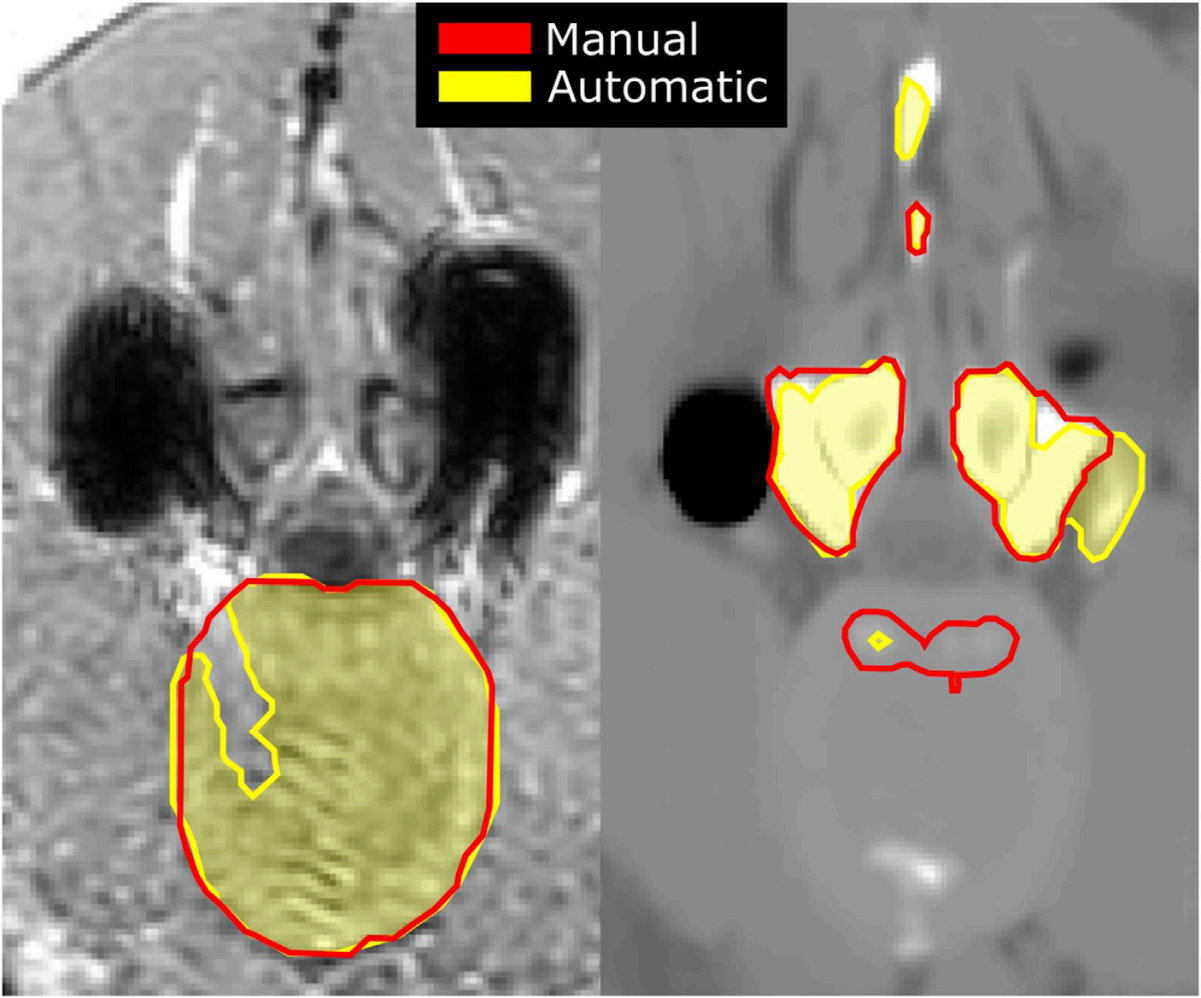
Artifacts originating from the spine implant on an acquired in-phase MR image (left), shown as dark signal voids, with manual (red) and automatic (shaded yellow) segmentations of the IVD; and an sCT image (right) of the same slice with artifacts originating from the signal voids leading to inaccurate segmentations of the vertebrae.
